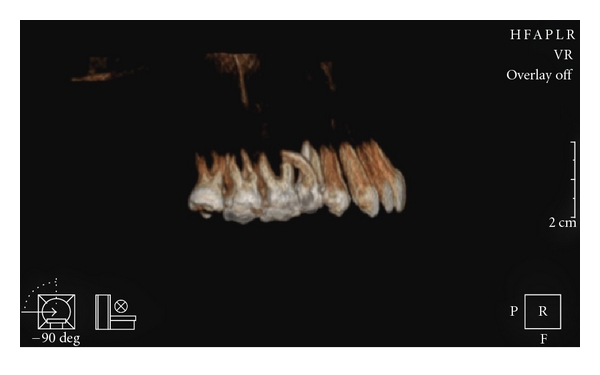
Reconstructed cone-beam computerized tomography image showing right side of the maxilla.
